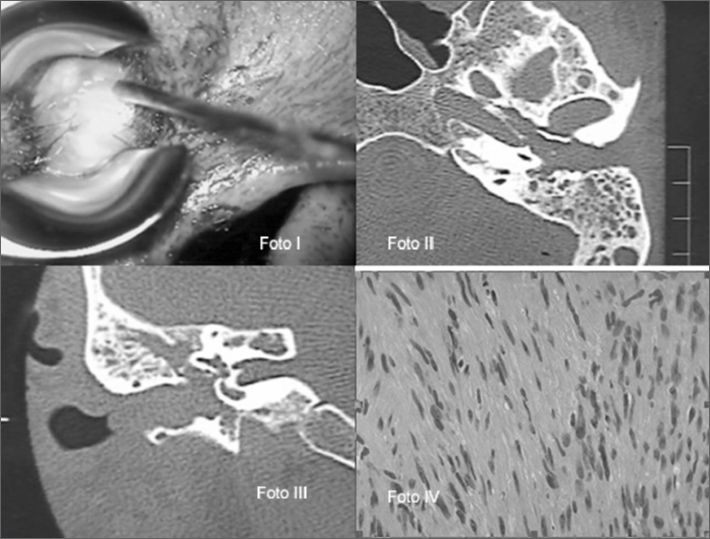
Photo. I: Polypoid tumor of the left ear coming out of the EEC. Photos II and III: Contrasted ear CT scan, axial and coronal views, showing lesion extension. Photo IV: Pathology study of the patient with middle ear schwannoma. Proliferation of spindle-shaped cells without atypia, suggestive of schwannoma.
